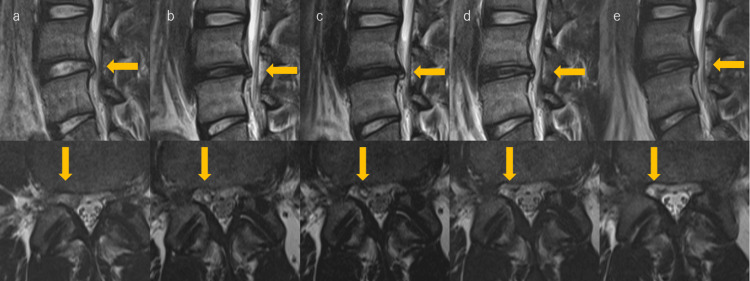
Course of L4/L5 disc herniation in the sagittal and horizontal sections of MRI (a) Preinjection, (b) four weeks after injection, (c) 13 weeks after injection, (d) 26 weeks after injection, and (e) 52 weeks after injection.
